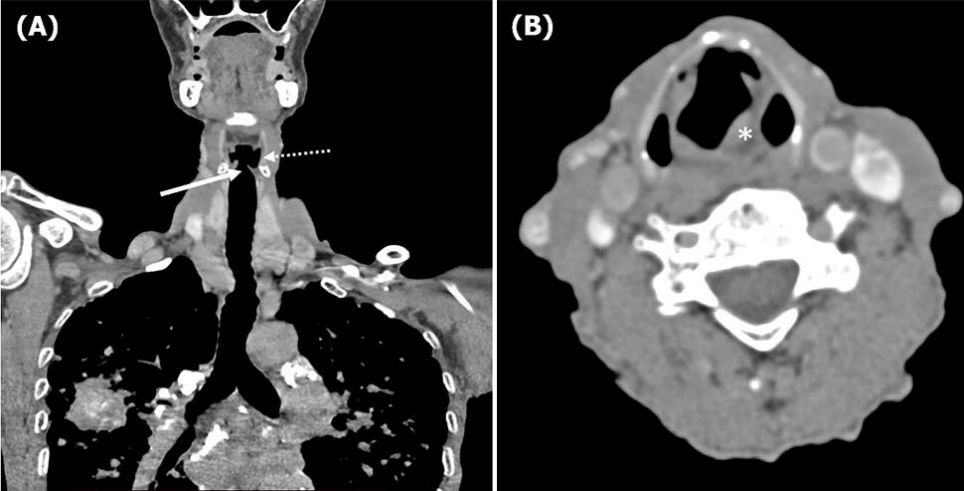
chest computed tomography scan in mediastinal window on coronal (A) and axial (B) views revealing signs of left vocal cord paralysis, most notably medialization and thickening of the aryepiglottic fold (asterisk), along with enlargement of the laryngeal ventricle “sail sign” (dotted and solid arrows)
